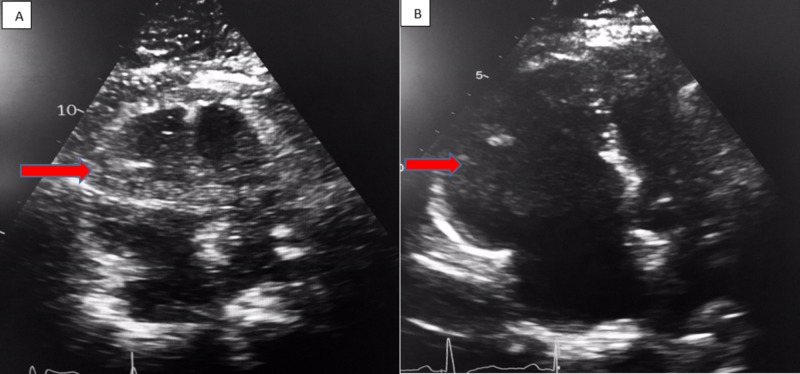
Apical four chamber view on transthoracic echocardiogram. (A) Shows increased RV:LV ratio (red arrow) in a patient with pulmonary embolism. (B) Shows increased RV:LV ratio of 1.8 (red arrow) in a patient with pulmonary embolism. RV: Right ventricular; LV: Left ventricular.
